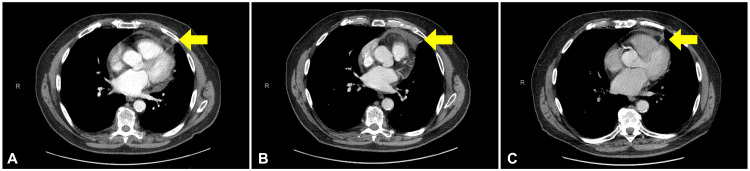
Follow-up chest CT imaging post-SABR at (A) 2.3 months, (B) 8.9 months, and (C) 40.1 months. CT = computed tomography, SABR = stereotactic ablative radiotherapy
